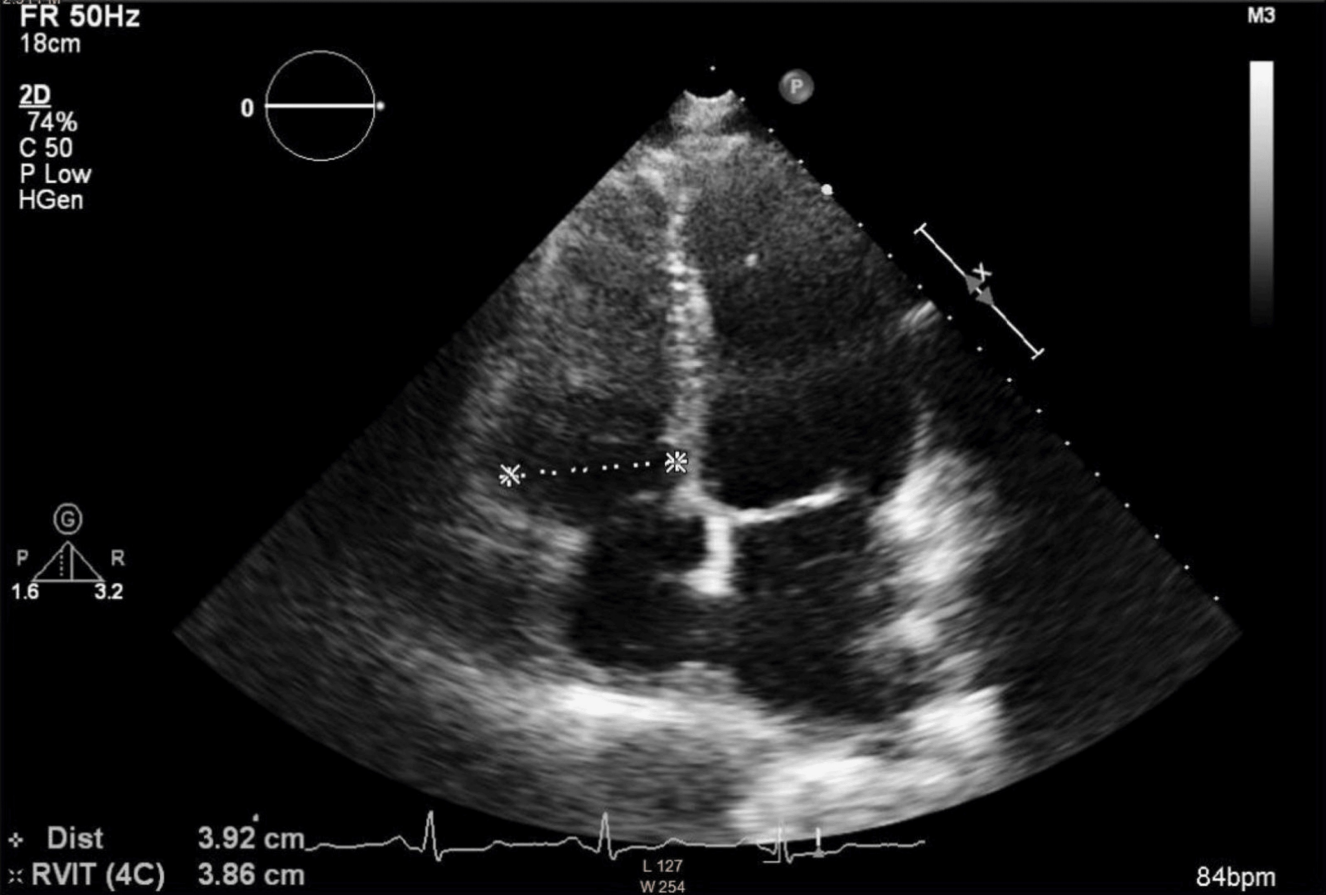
Right ventricle at the upper limit of normal size, assessed in the apical four-chamber view
